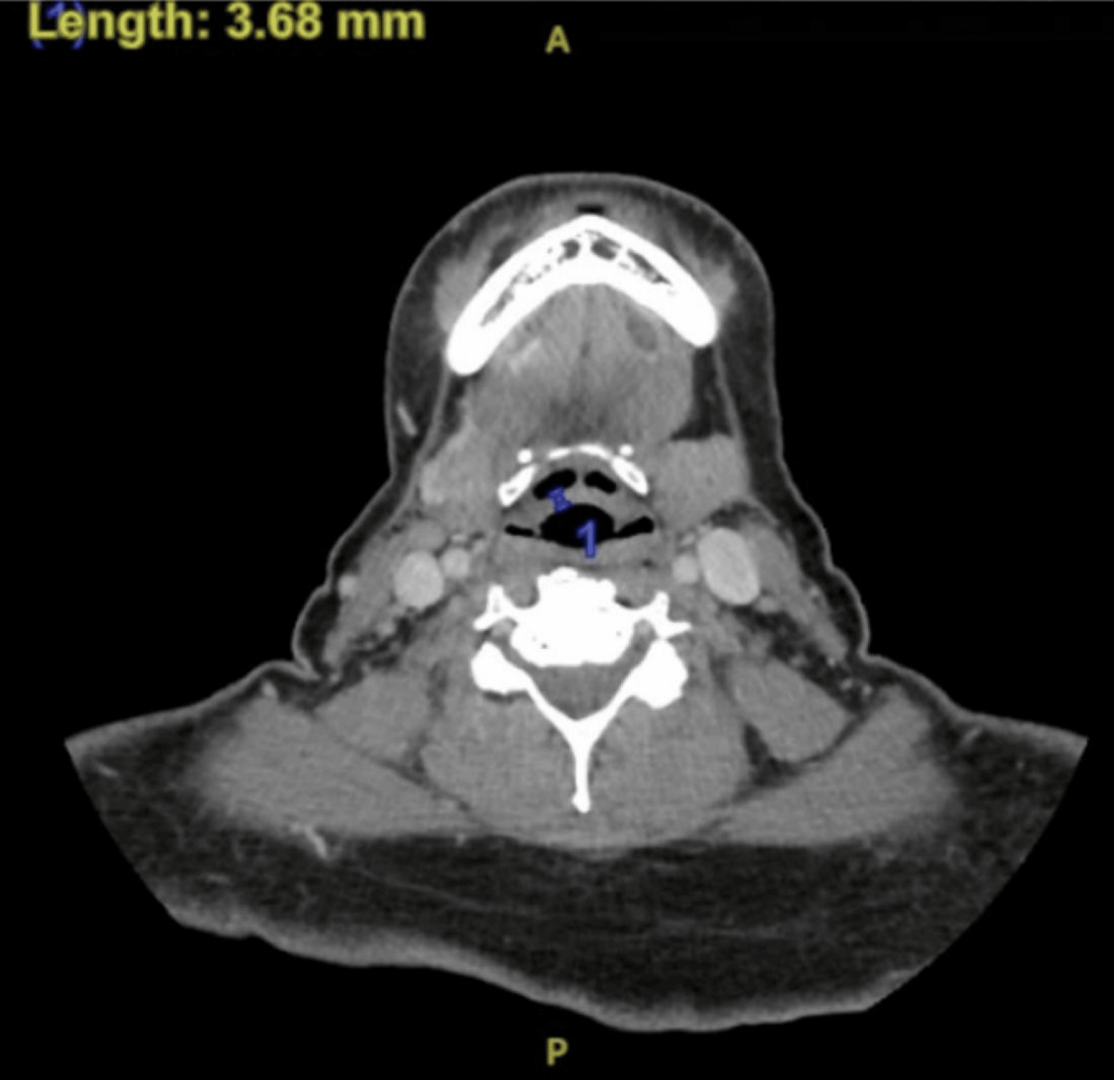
Axial CT of the Infrahyoid Neck Showing Mild Diffuse Epiglottic Thickening Measuring 3.68 mm, Consistent with Supraglottitis
